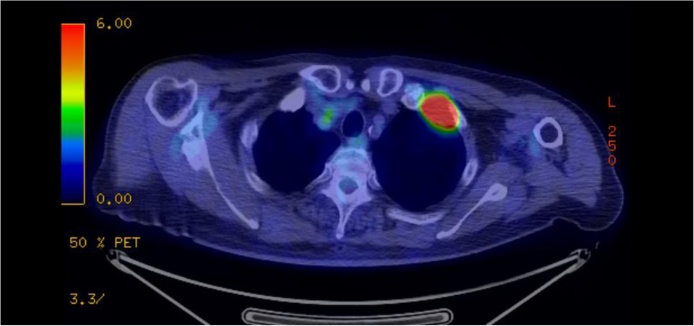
18-fluorodeoxyglucose (FDG) positron emission tomography/computed tomography showed a high FDG uptake within the tumor (SUV_max_: 12).
